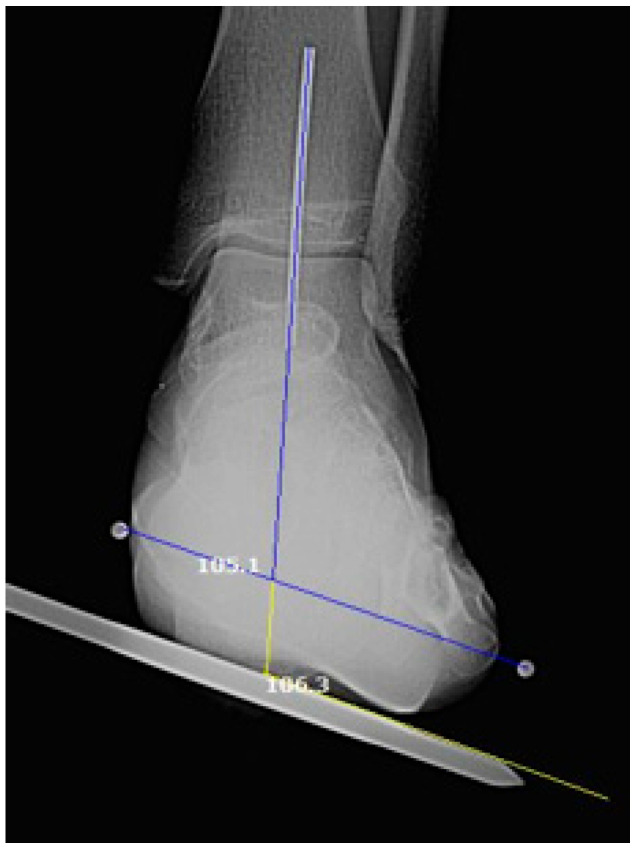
Radiographic assessment with the markers and nail.
